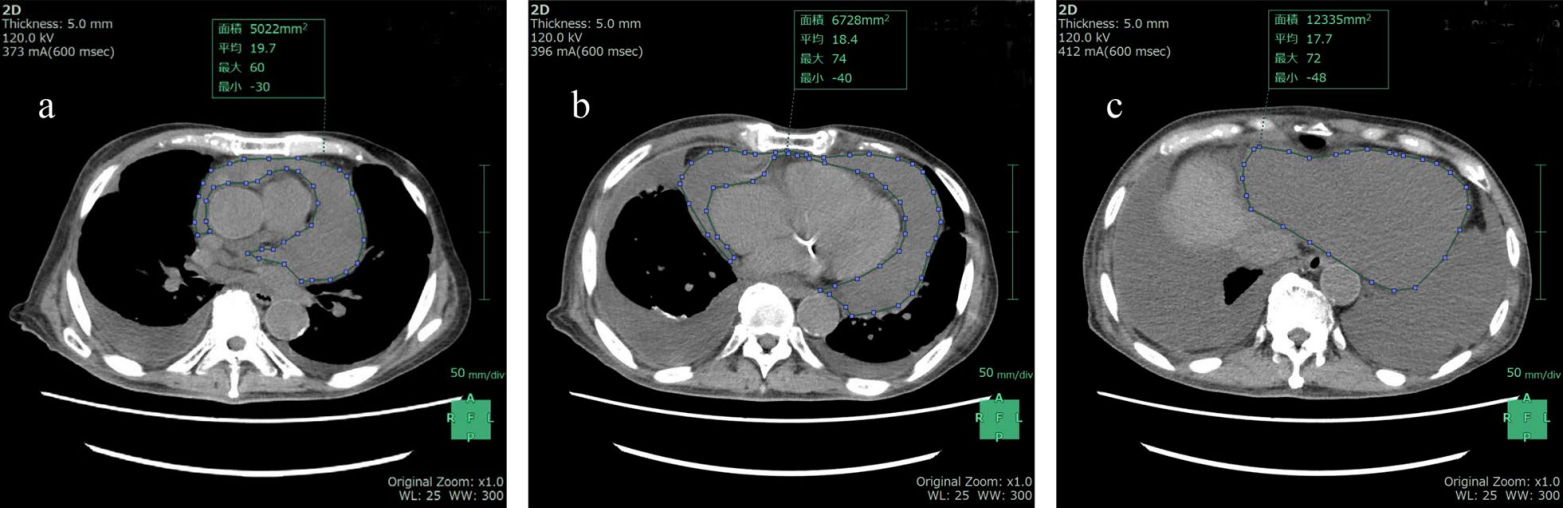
Measuring CT attenuation values in pericardial effusion. Two-dimensional images in three axial views: a upper slice: bifurcation of the pulmonary trunk, b middle slice: four-chamber heart view, c lower slice: upper edge of the liver. Both computed tomography (CT) attenuation values (in Hounsfield units (HU)) and pericardial effusion area were measured by plotting around the pericardial effusion in the three axial slices. The respective results were a 5022 mm^2^, 19.7 HU; b 6728 mm^2^, 18.4 HU; and c 12,335 mm^2^, 17.7 HU
